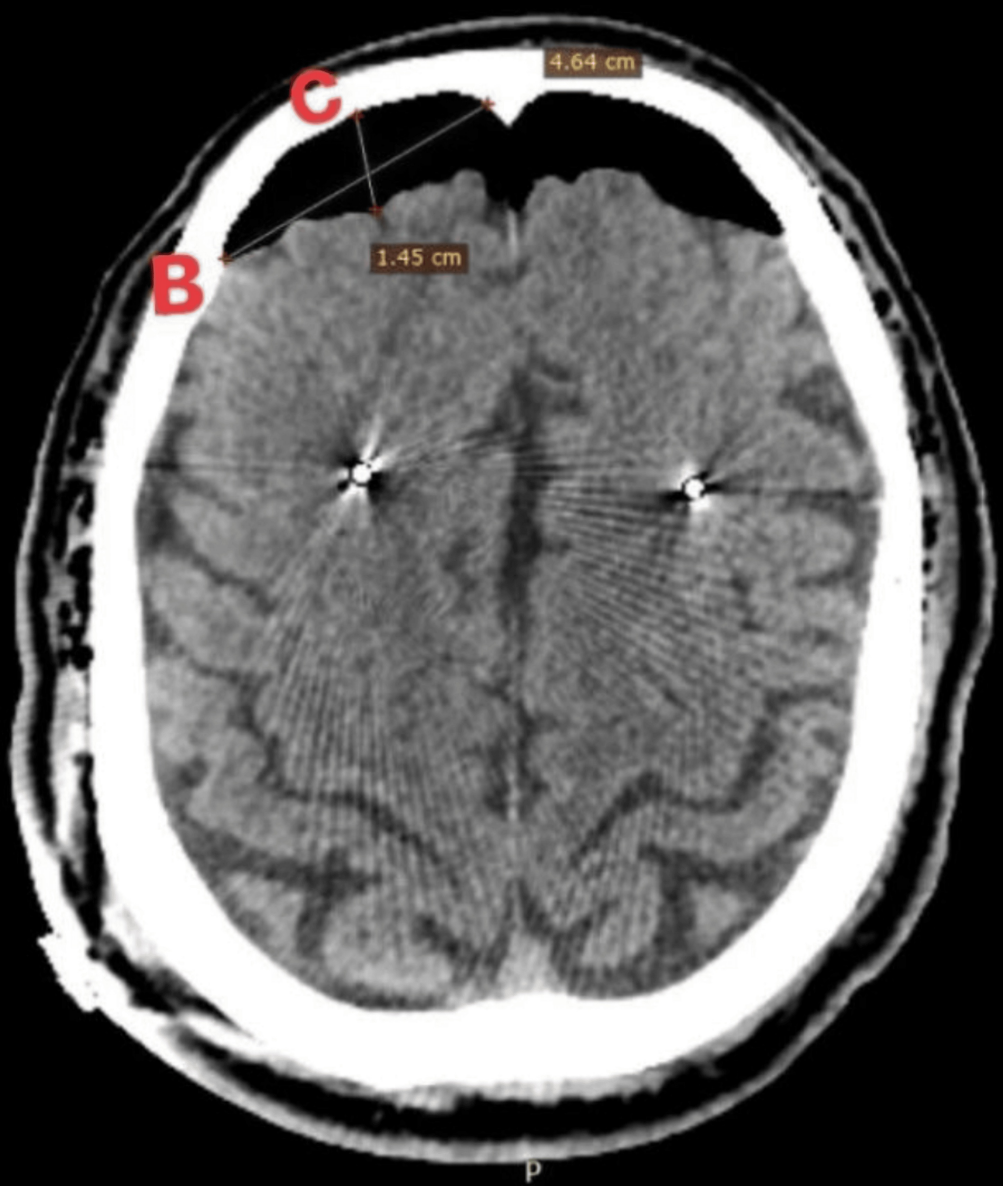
Early post-operative CT scan without contrast, showing subdural pneumocephalus of 25.11 cm³ A: the number of air pockets noted x section thickness, B: widest diameter in axial section, C: widest diameter perpendicular to B.
